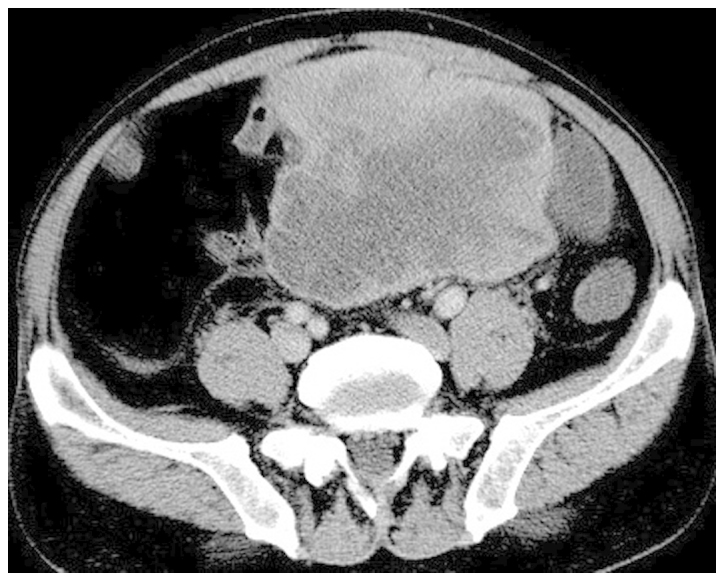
Computed tomography revealing heterogeneous enhancement with hypodense areas in the globular non-adipose tissue.
